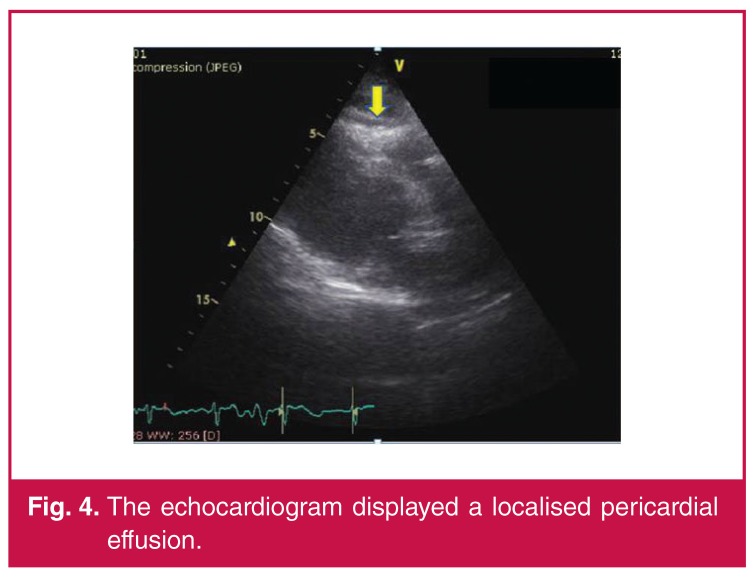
The echocardiogram displayed a localised pericardial effusion.
